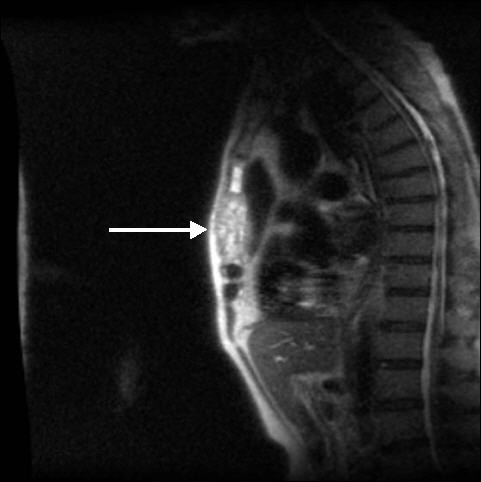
MRI scan demonstrating sternal metastasis with loss of marrow signal and abnormal cortical margins. The white arrow identifies the metastasis within the body of the sternum.
